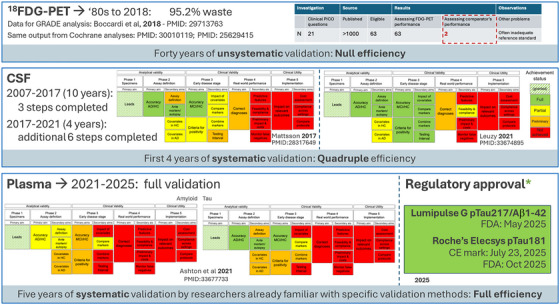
Efficiency of AD biomarker validation increased with availability and compliance with specific systematic methodology. AD, Alzheimer's disease; CE, Conformité Européenne, CSF, cerebrospinal fluid; FDA, US Food and Drug Administration; FDG, fluorodeoxyglucose; GRADE, Grading of Recommendations Assessment, Development and Evaluation; HC, healthy control; MCI, mild cognitive impairment; PET, positron emission tomography; PICO, Population, Intervention/Index test, Comparison, Outcome; ptau, phosphorylated tau
